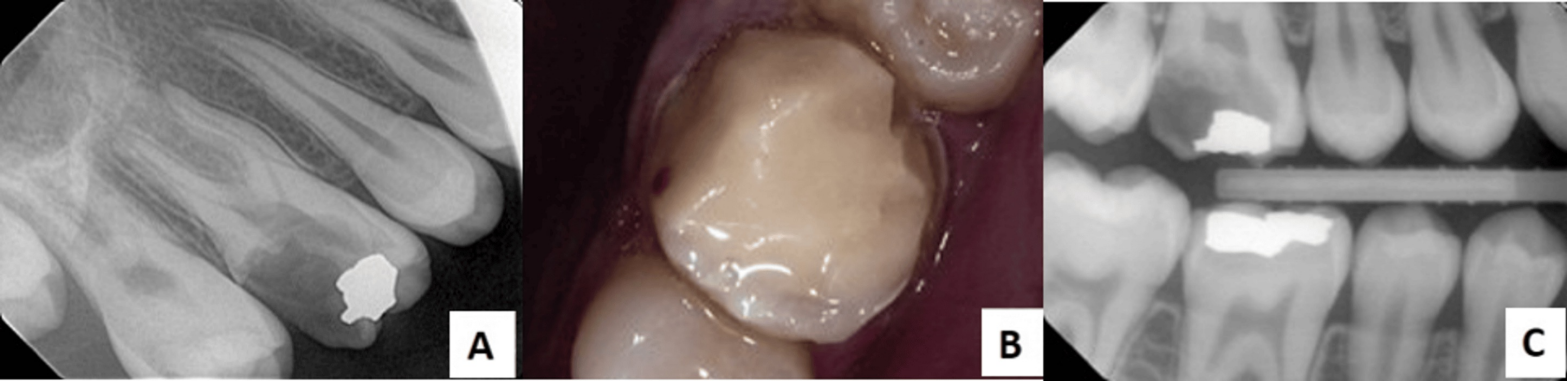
(A) Preoperative periapical radiograph; (B) Preoperative intraoral view with GIC restoration; (C) Preoperative bitewing radiograph. GIC: glass ionomer cement
